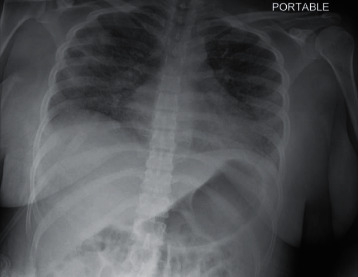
Portable chest X-ray film from our patient on day 5 of admission showing bilateral multifocal airspace opacities consistent with pneumonitis.
